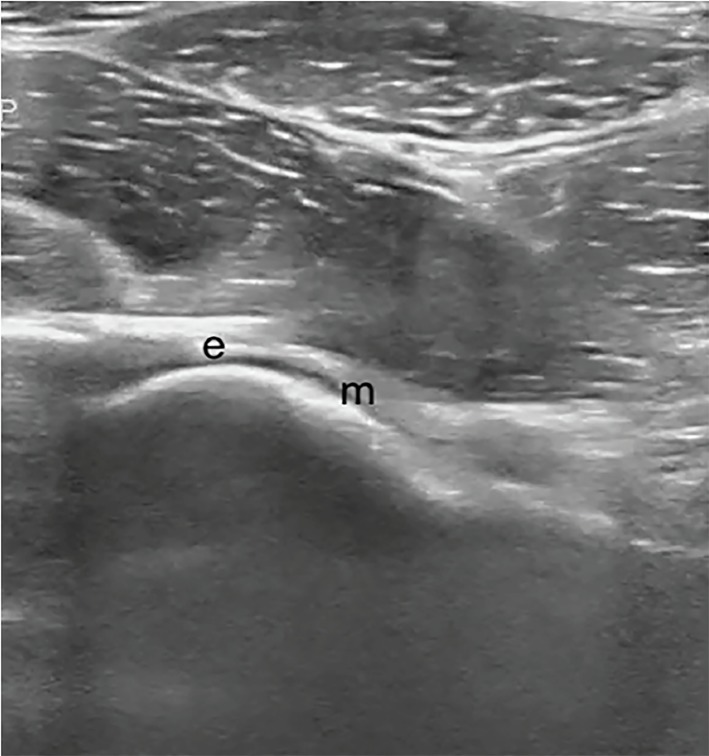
Point-of-care ultrasound image of right hip showing normal contour of metaphysis (m) and epiphysis (e) with no displacement.
